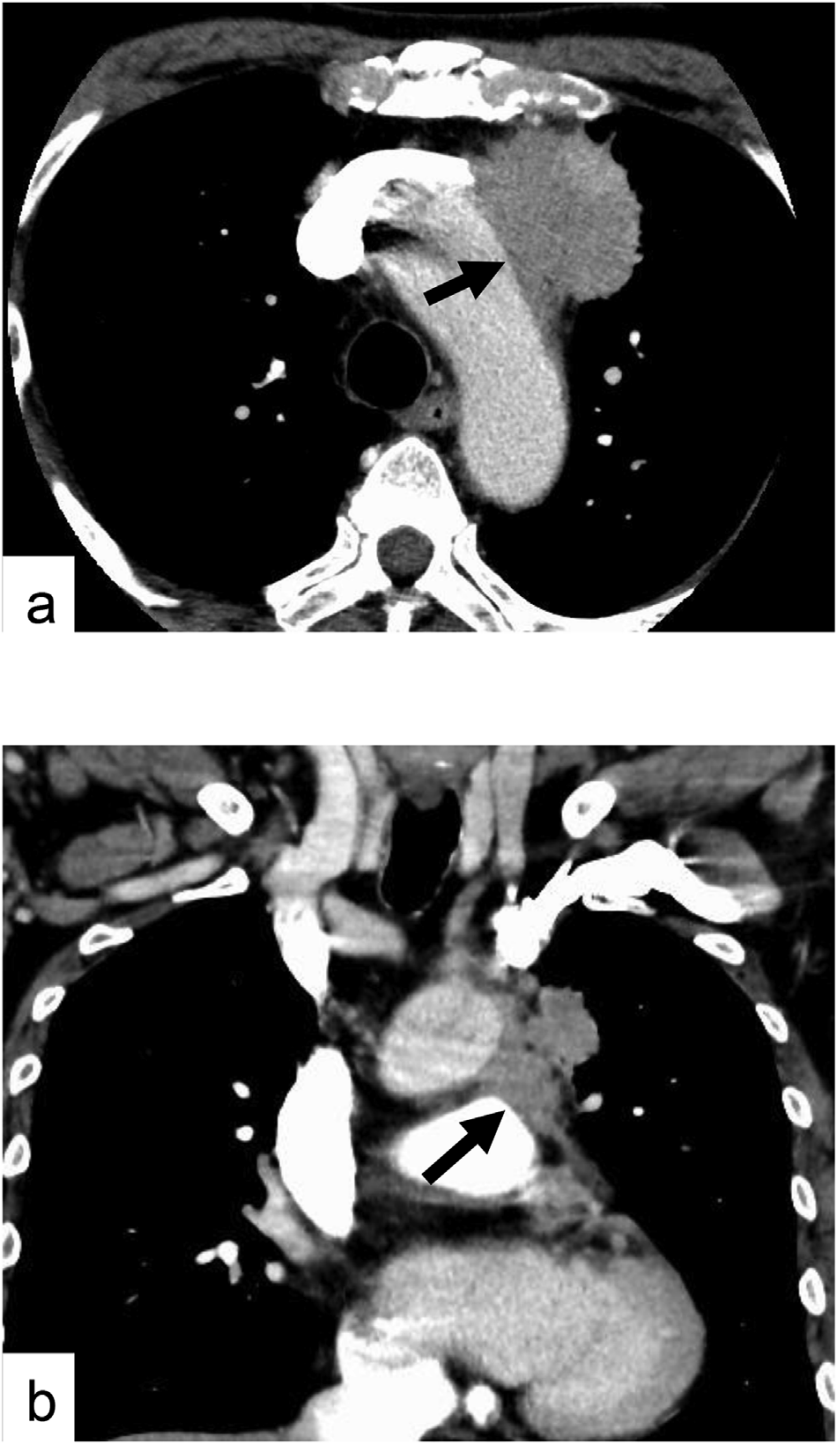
Computed tomography of the chest showing localization of the tumor in the anterior mediastinum. (a) Axial view showing that the tumor touches the aortic arch (arrow). (b) Coronal view showing that the tumor touches the main pulmonary artery (arrow).
